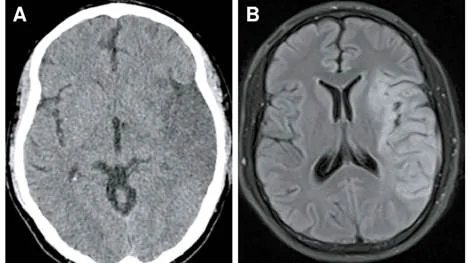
A) Tomografía simple de cráneo, ventana de cerebro, imagen axial a nivel de los tálamos. Se evidencia lesión hipodensa en el territorio de la arteria cerebral media del lado izquierdo, con pérdida de la diferenciación corticosubcortical y borramiento de surcos cerebrales, por edema citotóxico. No hay desplazamiento de la línea media. B) Resonancia simple de cerebro, secuencia FLAIR, imagen axial a nivel de los núcleos de la base. Se evidencia área hiperintensa en el territorio de la arteria cerebral media del lado izquierdo, comprometiendo la ínsula, el giro precentral, el giro poscentral y el lóbulo parietal inferior. La lesión se extiende a la sustancia blanca profunda y el brazo anterior de la cápsula interna. Hay leve efecto de masa con desplazamiento de la línea media.
